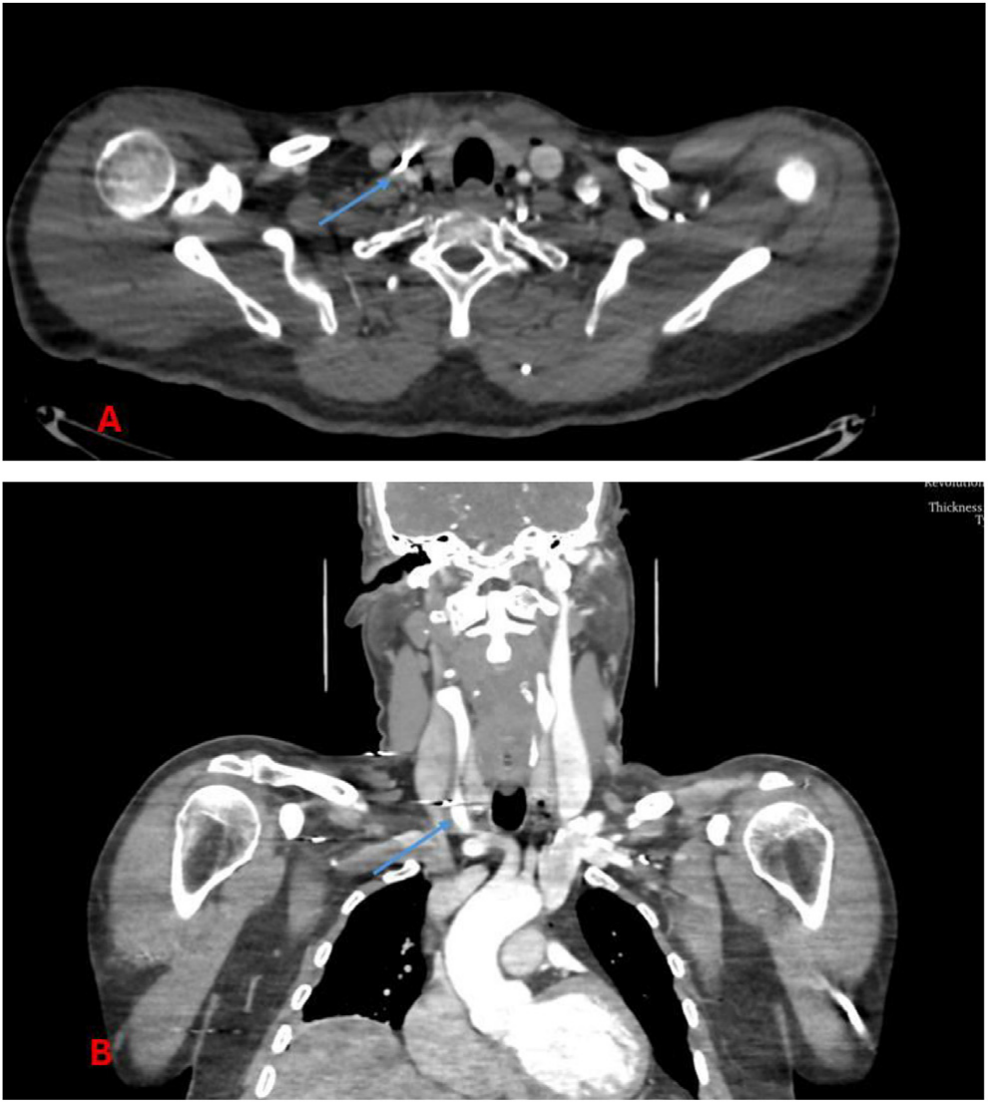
CTA image of the neck demonstrating a metallic pellet within the right common carotid sheath (arrow), without evidence of luminal narrowing or contrast extravasation. (A) axial view; (B) coronal view.•Pretracheal, in contact with the right thyroid lobe, without visible tracheal breach (Fig. 5).
